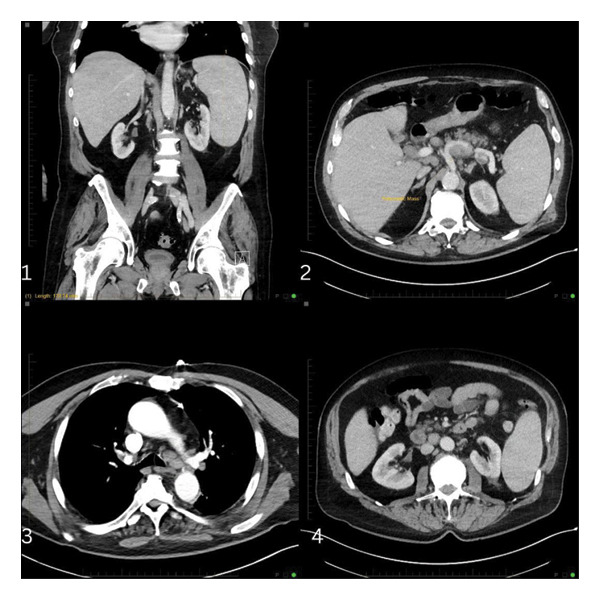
CT chest, abdomen, and pelvis with contrast at presentation revealed (image 1) splenomegaly measuring up to 17 cm in craniocaudal dimension, (image 2) a 4 cm nodular density within the pancreatic tail abutting the left splenic artery, (image 3) extensive lymphadenopathy including bilateral hilar and mediastinal nodes, and (image 4) additional lymph nodes in the porta hepatis measuring up to 21 mm.
